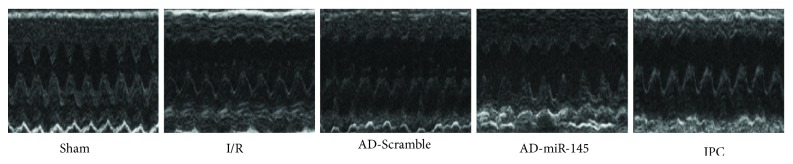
Representative images of echocardiograph.
